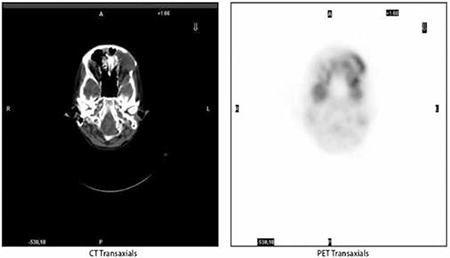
Left orbital involvement of the disease in axial CT and FDG accumulation corresponding to the soft tissue involvement in axial PET image respectively
